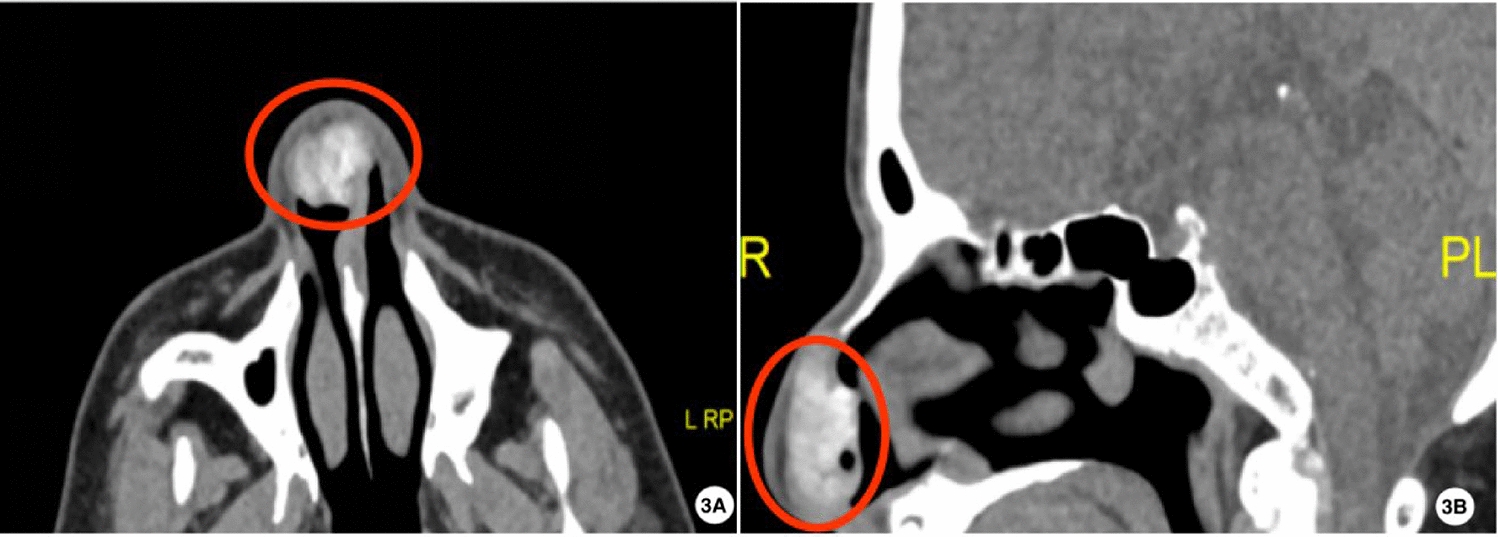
Axial computed tomography of nasal cavity (A) and sagittal computed tomography of nasal cavity (B). Soft tissue nodule is observed in the anterior nasal septum, protruding into the right nasal vestibule. The nodule exhibits irregular calcification (circled). The lesion is well demarcated, measuring approximately 16 mm × 20 mm × 27 mm. The nodule’s attenuation values ranged from 110 to 220 Hounsfield units
